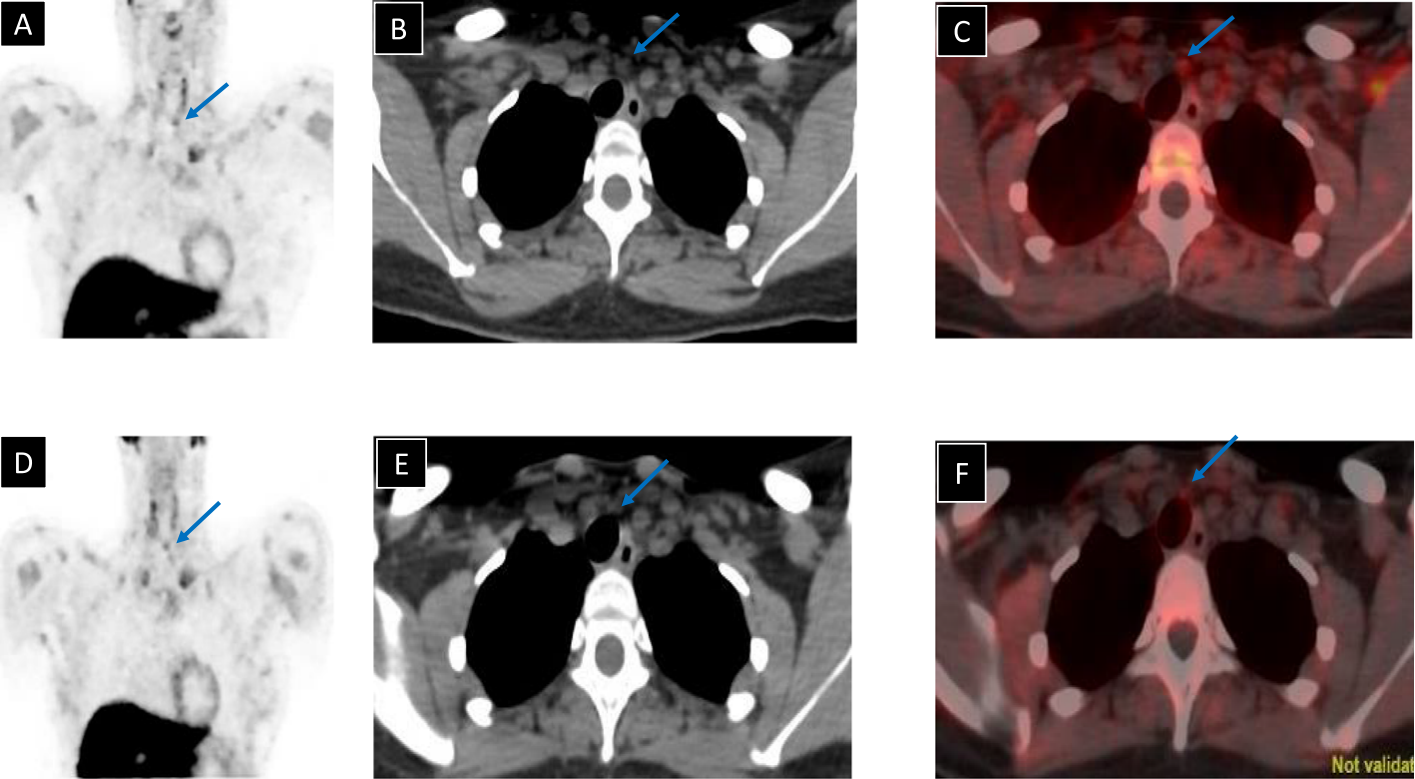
^18^F-fluoroholine PET/CT. Images were obtained before (a, b, and c) and after (d, e, and f) left inferior parathyroidectomy. Coronal PET (a and d), axial CT (b and e), and fused PET/CT (c and f) images show choline-avid lesion inferior to the lower pole of the left thyroid lobe (blue arrows) that persisted after parathyroidectomy
